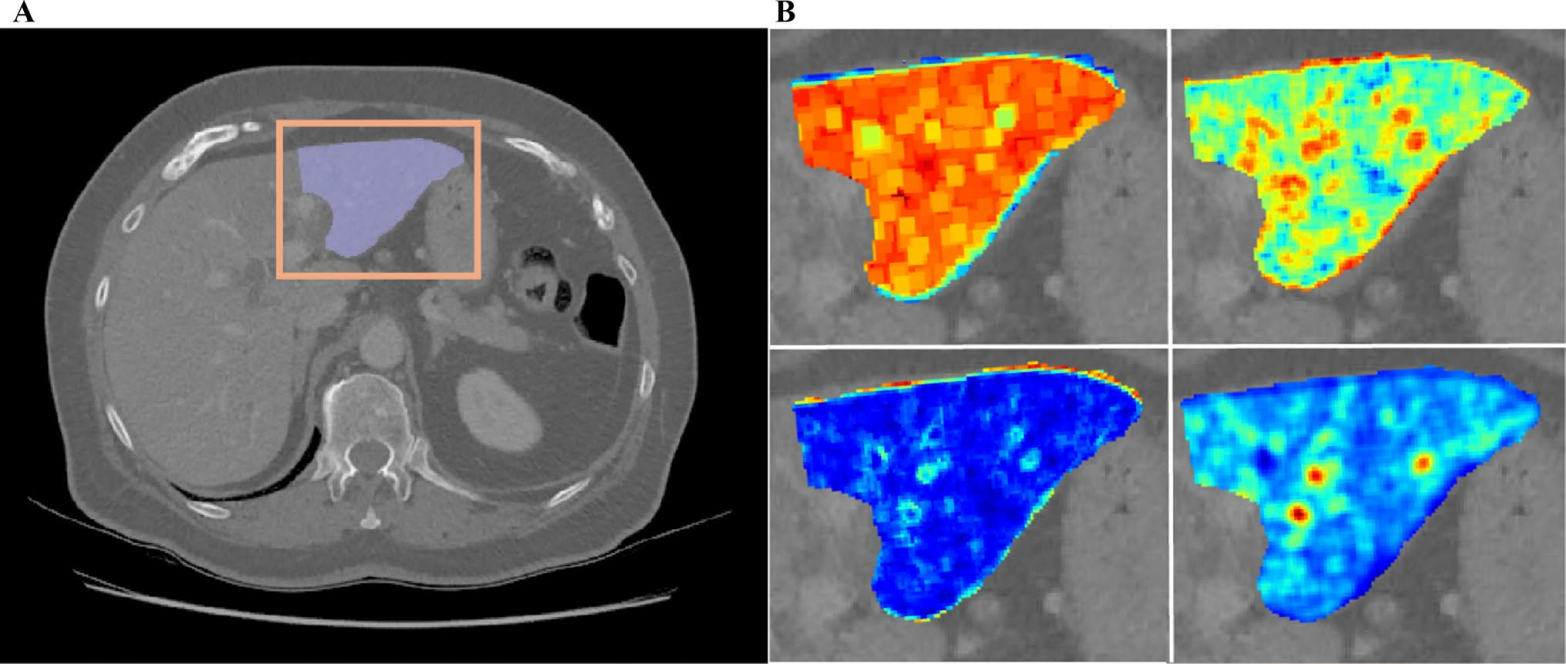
A representative case of CT study. A Radiomics features were extracted from the segmented future liver remnant part at the slice of the bifurcation level of the main portal vein. B Four representative radiomics features that show a texture pattern different from that perceived by human naked eye
